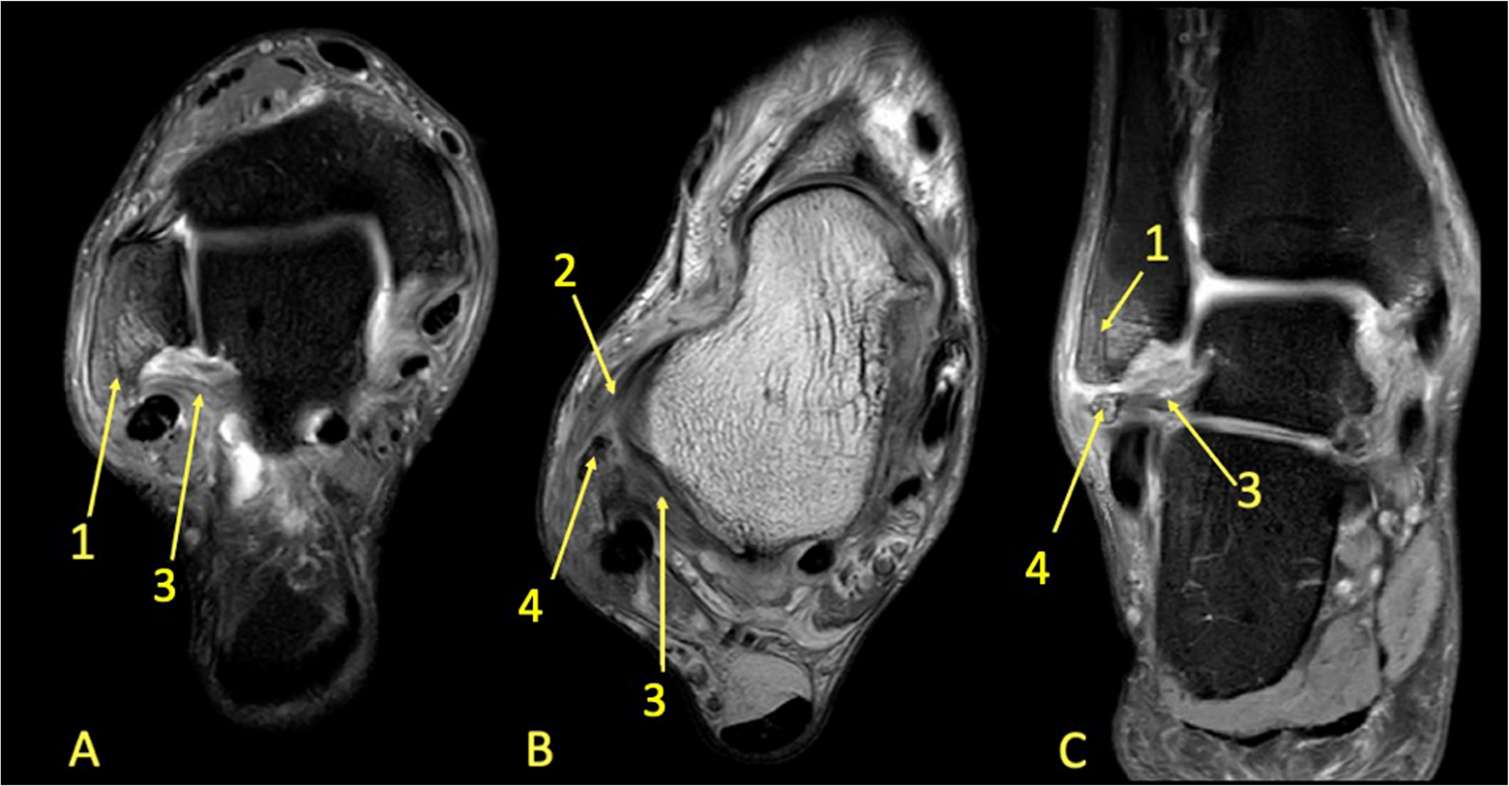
A 24-year-old male imaged on suspicion of an anterior tibiofibular ligament rupture 2 weeks after an ankle injury. MRI showed an avulsion of the anterior talofibular ligament. (1) BME in the lateral malleolus, (2) the anterior talofibular ligament, (3) the posterior talofibular ligament, and (4) an avulsed bone fragment from the lateral malleolus
